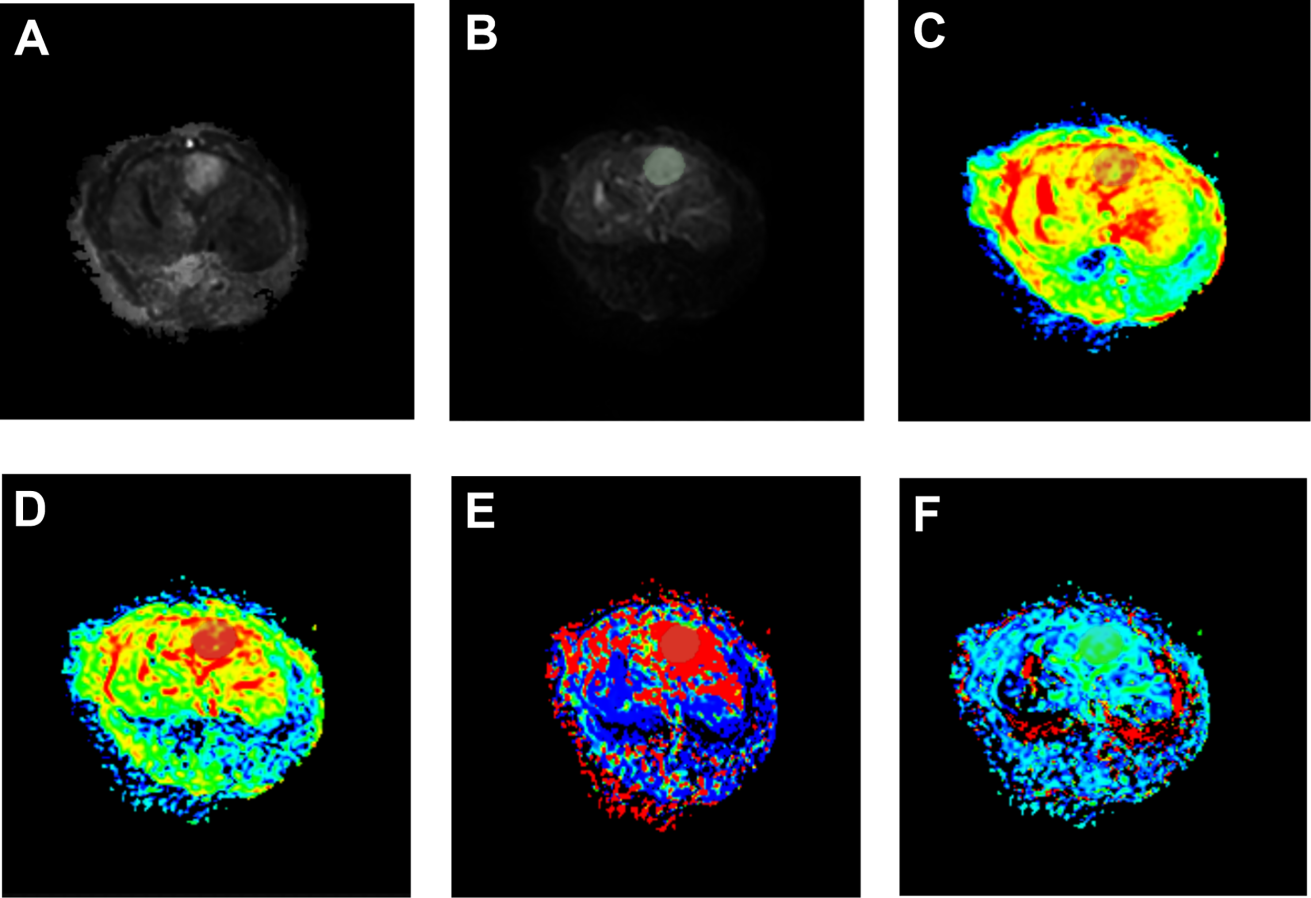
Pseudo-color images of IVIM-related parameters 7 days after TAE combined with apatinib treatment. The tumor showed a significantly high signal on raw DWI images (A), post-processed DWI images (B). In pseudo-color images of ADC (C), pseudo-color images of D (D), pseudo-color images of D* (E), and pseudo-color images of *f*
